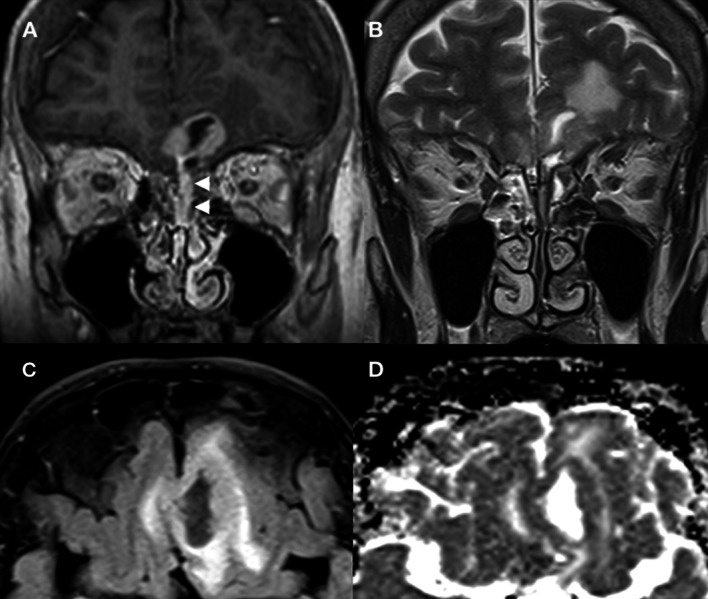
MRI of the anterior skull base. Coronal T1 post-contrast (a) and coronal T2 (b) demonstrate a mixed solid cystic mass extending from the olfactory recess of the left nasal cavity (white arrowheads) through the left cribriform plate and into the anterior cranial fossa. The mass is adhered to the pial surface of the frontal lobes of the brain bilaterally. There is adjacent vasogenic oedema within the brain parenchyma on the coronal T2 (b) and axial FLAIR (c) sequences. The solid portions demonstrate avid enhancement (a) and intermediate to restricted apparent diffusion (d) consistent with a cellular neoplasm
